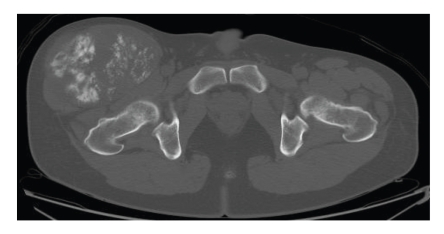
Computed tomography of thigh. The tumor was not in contact with the femur or its periosteum.
